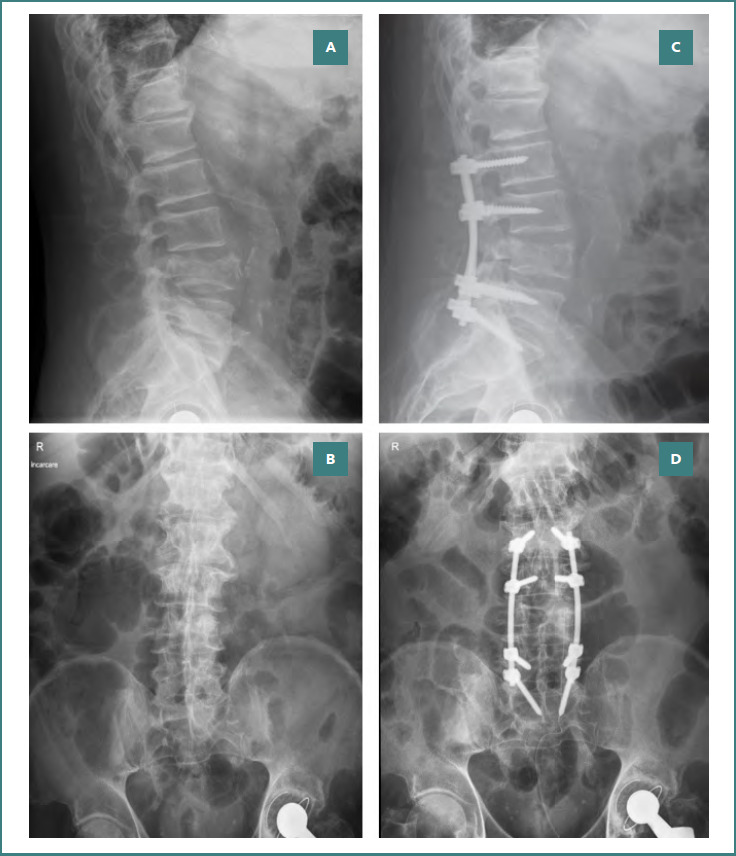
Preoperative and postoperative radiographs of a patient with L3–L4 spondylodiscitis treated by posterior decompression and L2–S1 fusion with pedicle screw instrumentation. A, Preoperative sagital view; B, Preoperative anteroposterior view; C, Postoperative sagital view; D, Postoperative anteroposterior view.
